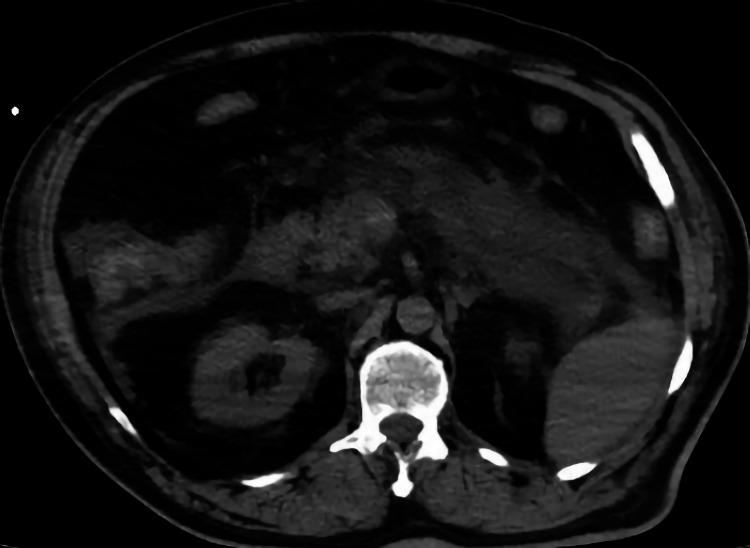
Increase in peripancreatic streaking, and fluid Non-contrast axial CT image showing an increase in peripancreatic streaking, and fluid CT: computed tomography
